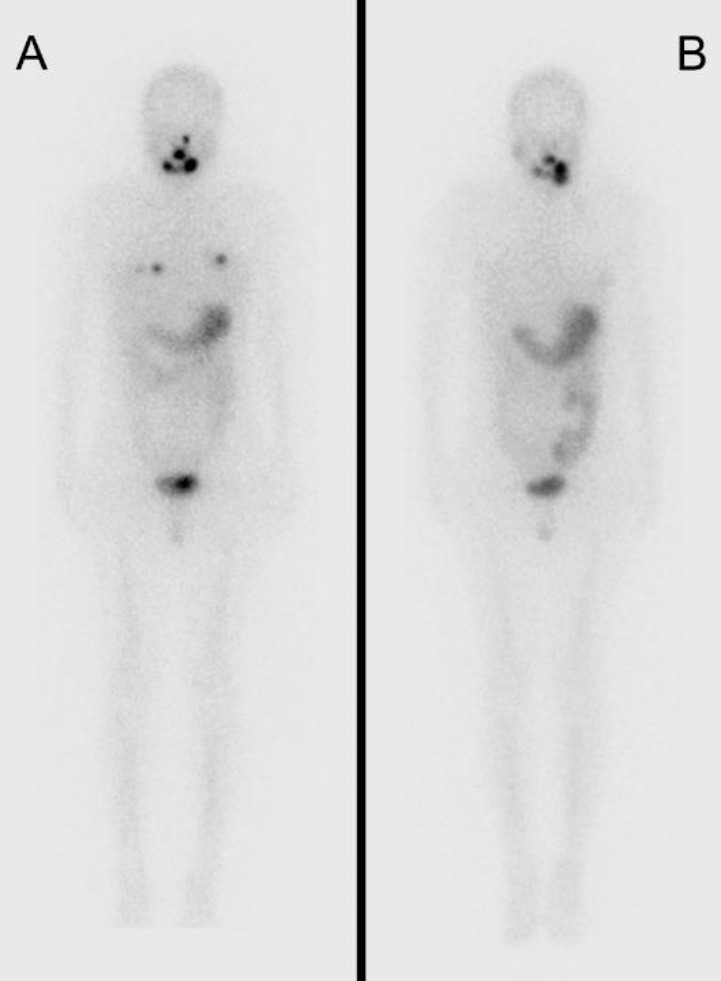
In the I-131 scintigraphy after the 2nd radioactive iodine therapy (RAIT) (A), accumulations have disappeared in the brain and shrunk in other regions. After the 3rd RAIT (B), accumulations have disappeared in all the region
